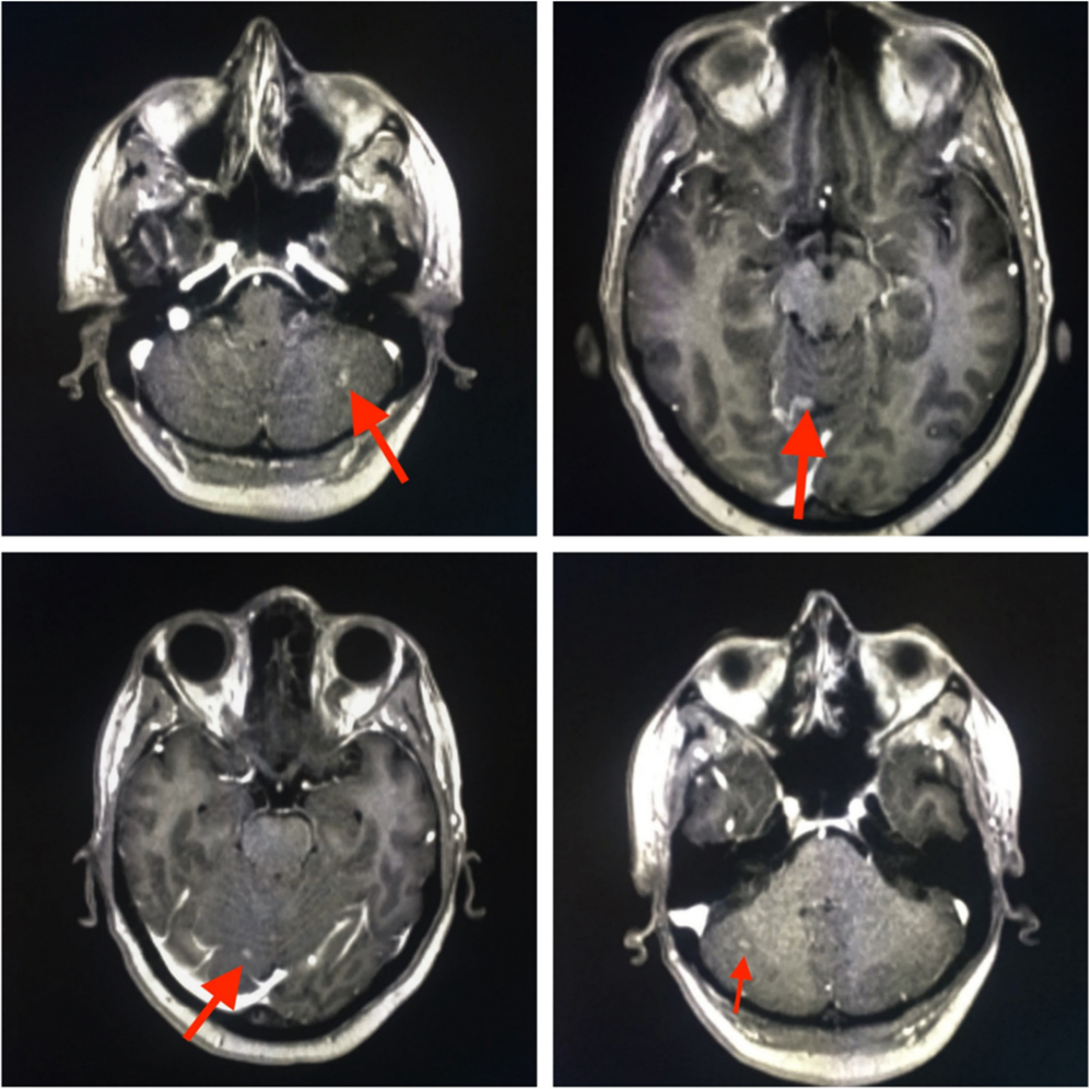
April 2016, Brain MRI showing multiple brain and leptomeningeal metastases
